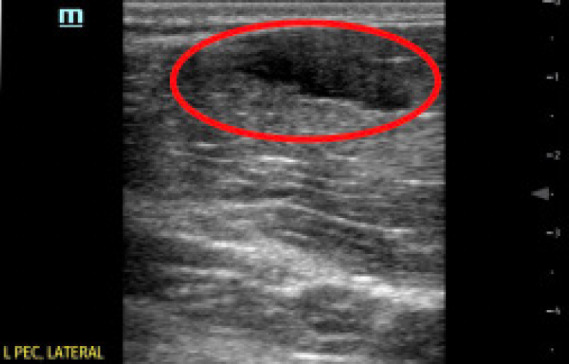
Point-of-care ultrasound image displaying hypoechoic hematoma formation (red circle) in the distal portion of pectoralis major muscle bellies.
